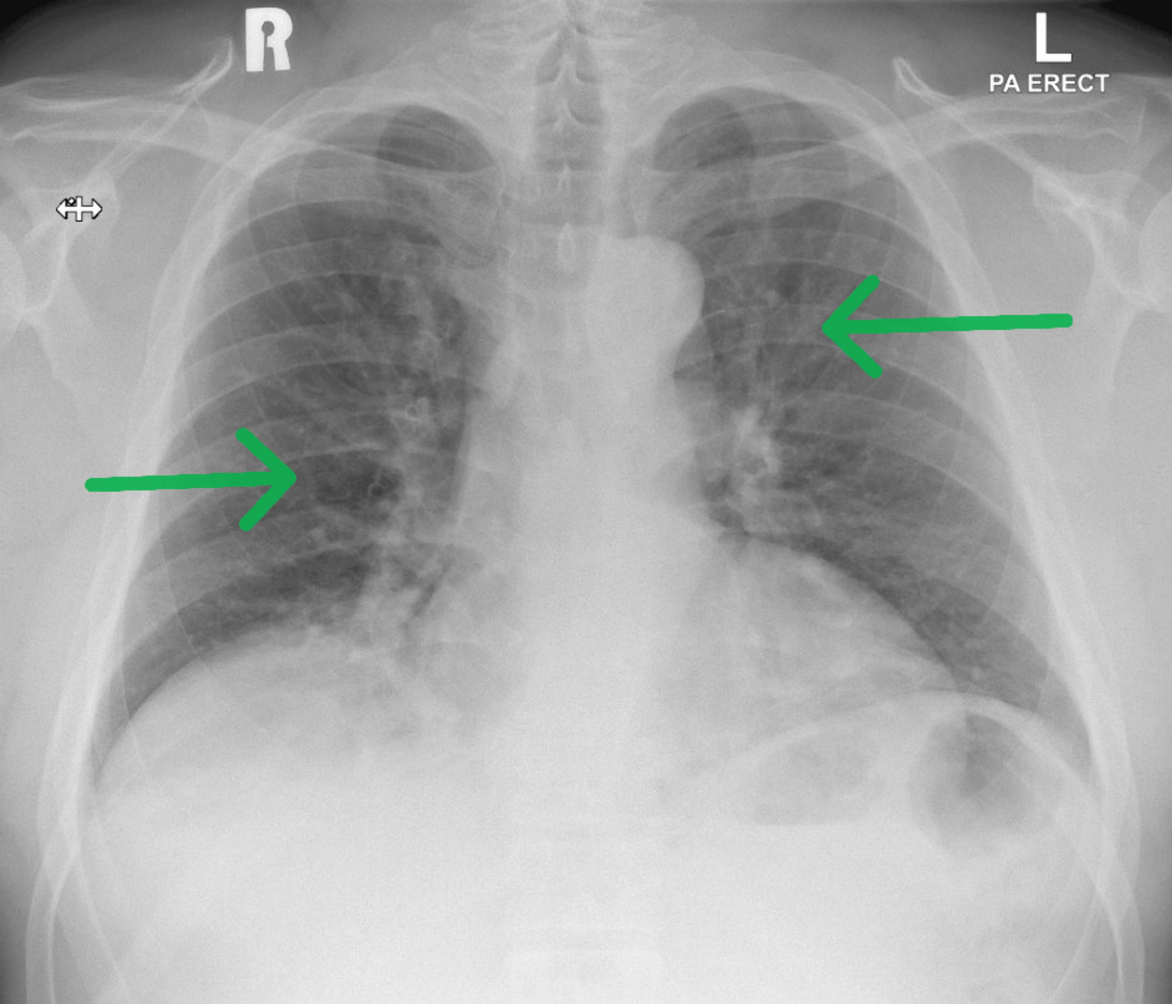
Chest X-ray showed increased bronchovascular markings
